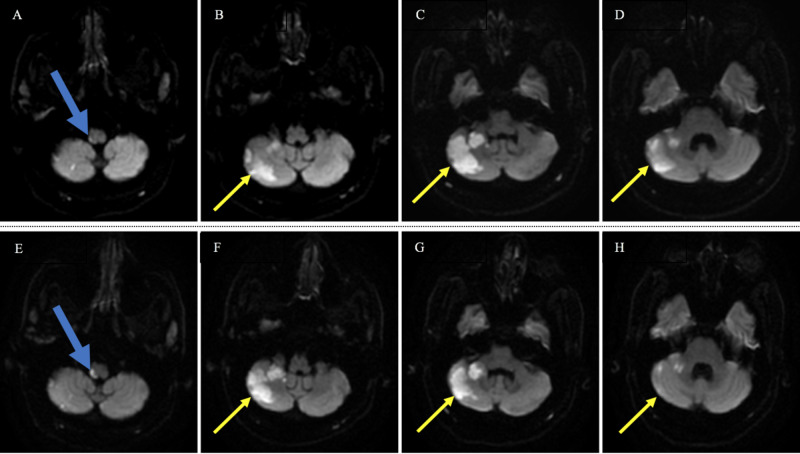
Initial and Secondary Stroke Images above and below each other correspond to the same anatomical plane. (A-D) Initial MRI: consecutive, rostral to caudal, axial DW MRI images with the blue arrow directed at hyperintensity representing small infarct in the midline-oriented area postrema; yellow arrows depict hyperintensity signifying the extent of full stroke extending from lateral medulla. (E-H) Repeat MRI: consecutive, rostral to caudal, axial DW MRI images with the blue arrow directed at hyperintensity representing worsening infarct in the region of area postrema; yellow arrows again represent regions of newly evolved stroke within the lateral medulla. DW, diffuse-weighted
